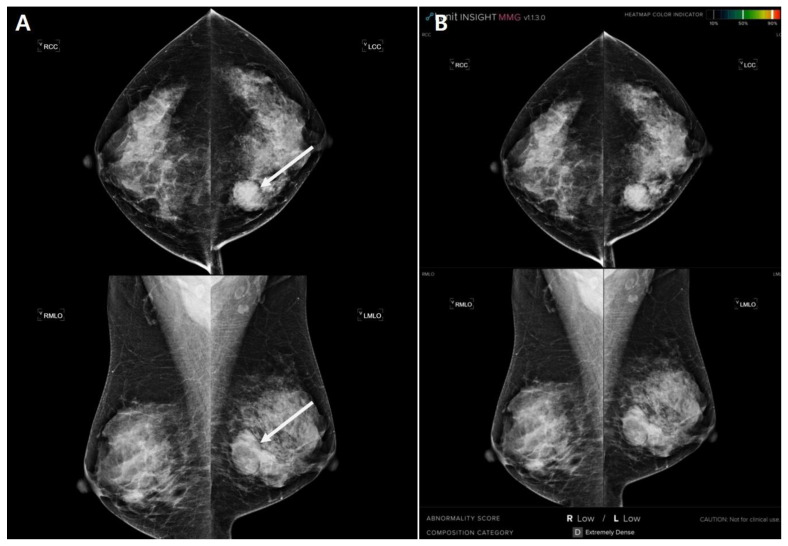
An invisible case in AI that is concordant only in radiologist’s analysis. A 53-year-old woman presented at the hospital with a palpable mass of her left breast. (A) Mammography showed a dense breast with a 3.7-cm-sized well-circumscribed oval mass in the left mediocentral breast (arrows). (B) Artificial intelligence revealed no abnormal lesion on the mammogram. The largest malignancy probability score was 0.85% of the left craniocaudal view and the abnormality score of the case was shown as ‘low’. The patient underwent ultrasound-guided core needle biopsy. She was diagnosed with invasive mucinous carcinoma.
